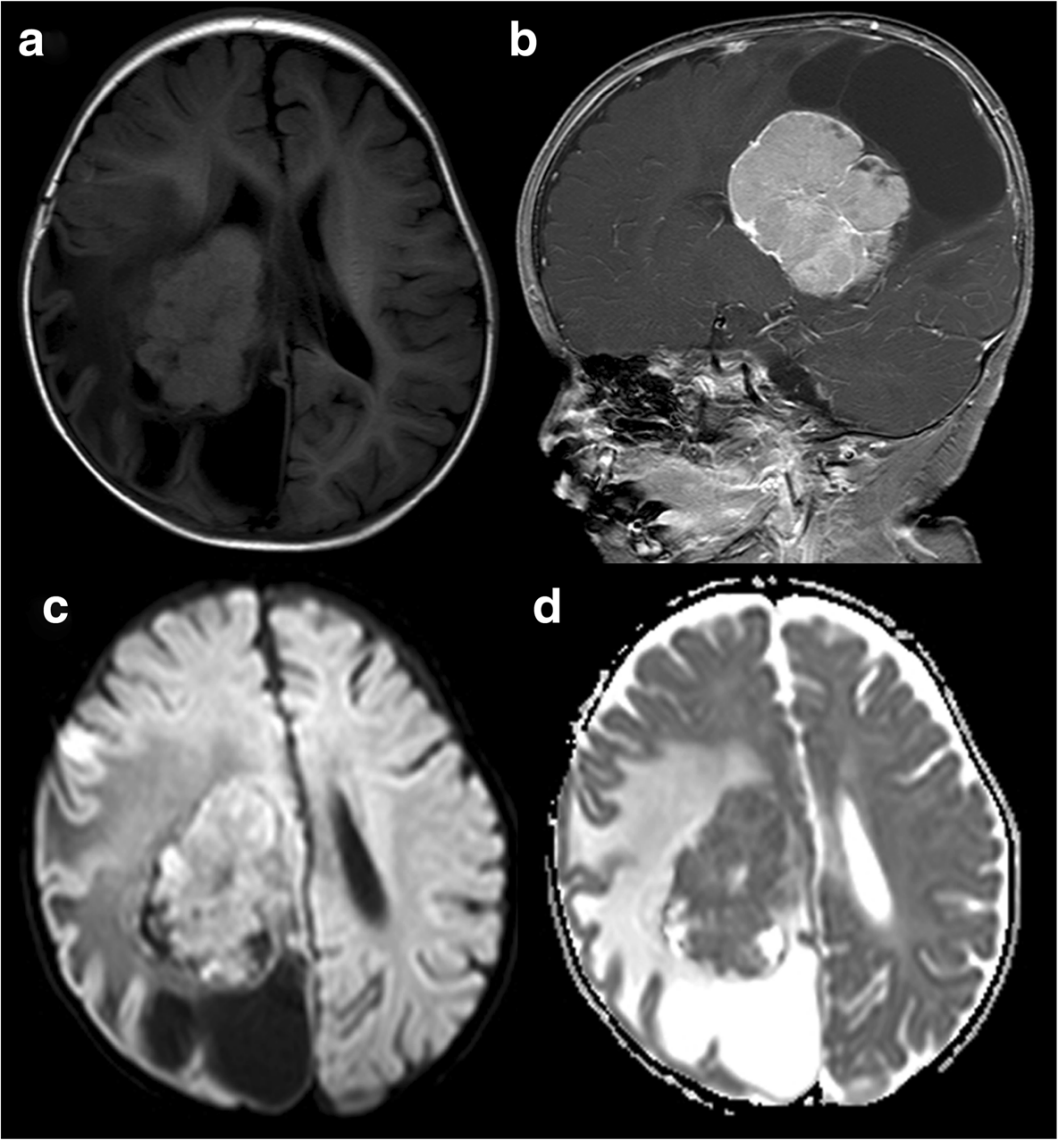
CPC (grade III). An 8-month-old girl with a lobulated lesion in the right lateral ventricular trigone. The solid part of the tumour is isointense on T1WI with multiple intratumoural necrosis and cysts. The surrounding brain parenchyma shows widely arranged oedema (a). Sagittal post-contrast imaging shows that the tumour is strongly enhanced (b). DWI sequence (c) reveals a hyperintense area, corresponding with a slightly hypointense area on the ADC map (d)
